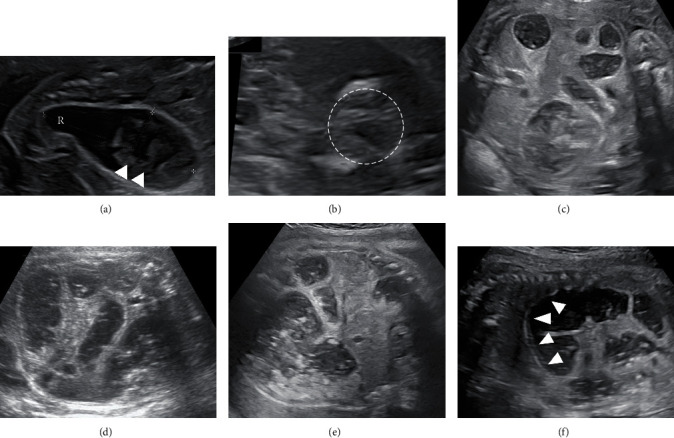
Fetal ultrasonography and the change of fetal bowel. Fetal images. (a) The distal rectal pouch shows dilated bowel with intraluminal calcifications (arrowheads) at 20 weeks. R indicates distal rectal pouch. The arrowheads indicate bowel calcifications. (b) The echogenic center (dotted circle) is not detectable at 20 weeks, which indicates that anal mucosa is invisible. (c) Fetal bowel dilation at 26 weeks. (d) Fetal bowel dilation at 31 weeks. (e) Fetal bowel dilation at 32 weeks. (f) Thoracic compression by bowel dilation at 31 weeks. The arrowheads indicate the diaphragm.
